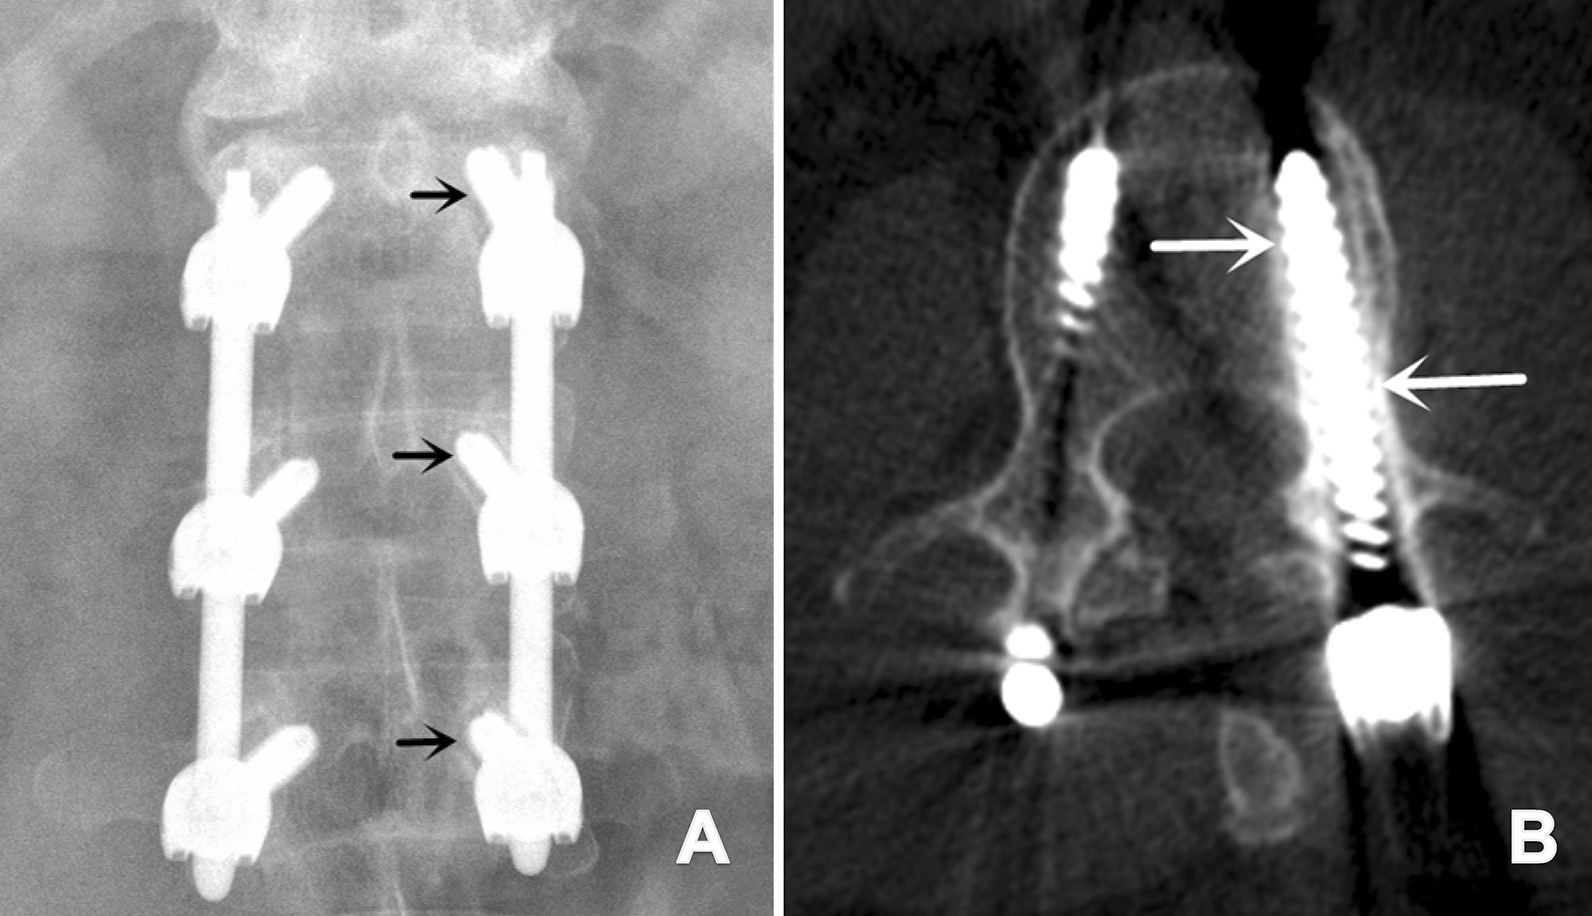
A Postoperative 1-year plain X-ray demonstrated left screw loosening at T12, L1, and L2 in a 39-year-old male who underwent short-segment fixation from T12 to L2 (black arrow: double halo sign); B postoperative 1-year CT demonstrated left screw loosening at L1 in a 47-year-old male who underwent short-segment fixation from T11 to L12 (white arrow: lucent zone)
